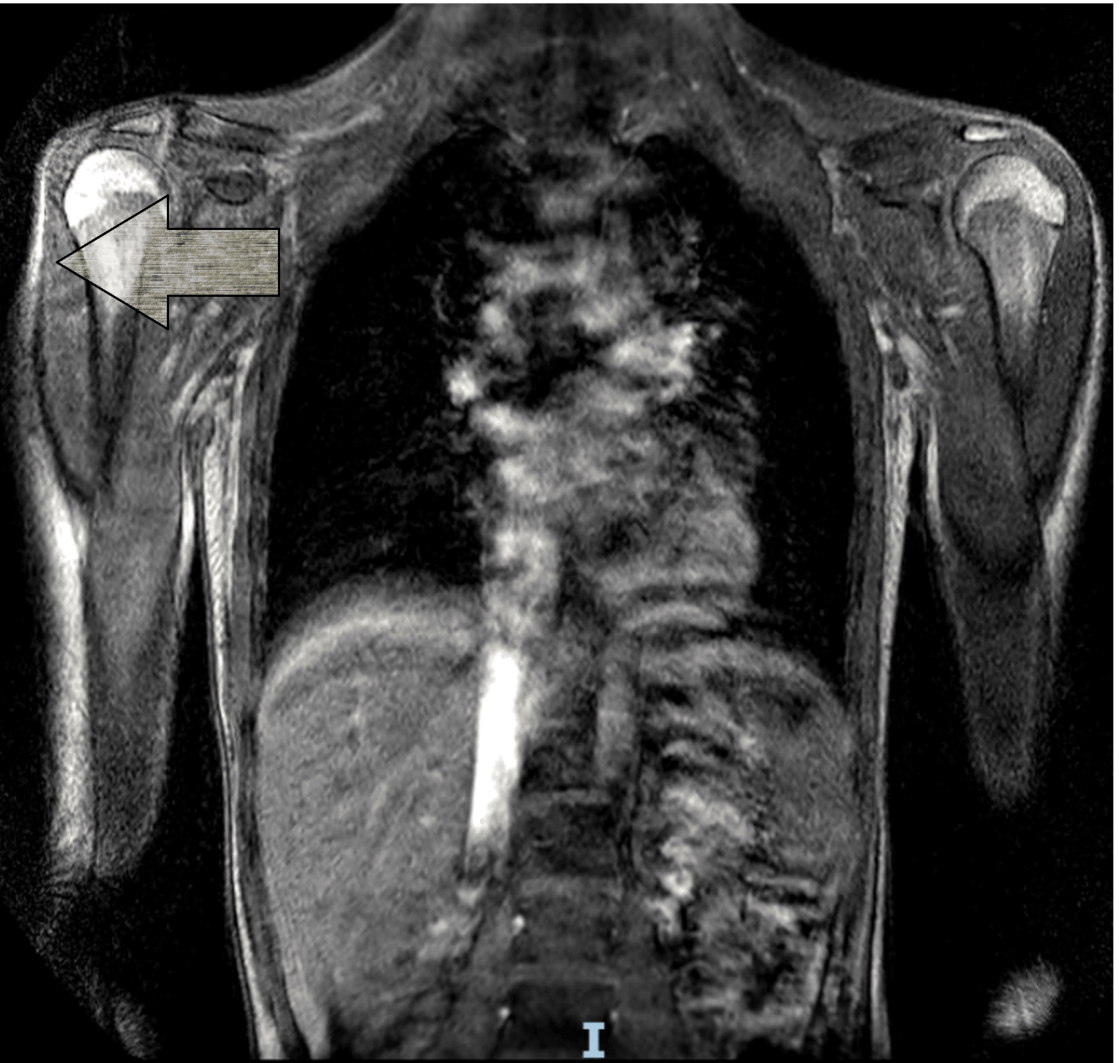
MRI scan of the thorax showing extensive dermatomyositis affecting the shoulder girdle and chest. Features of dermatomyositis are indicated by an arrow. MRI, magnetic resonance imaging
